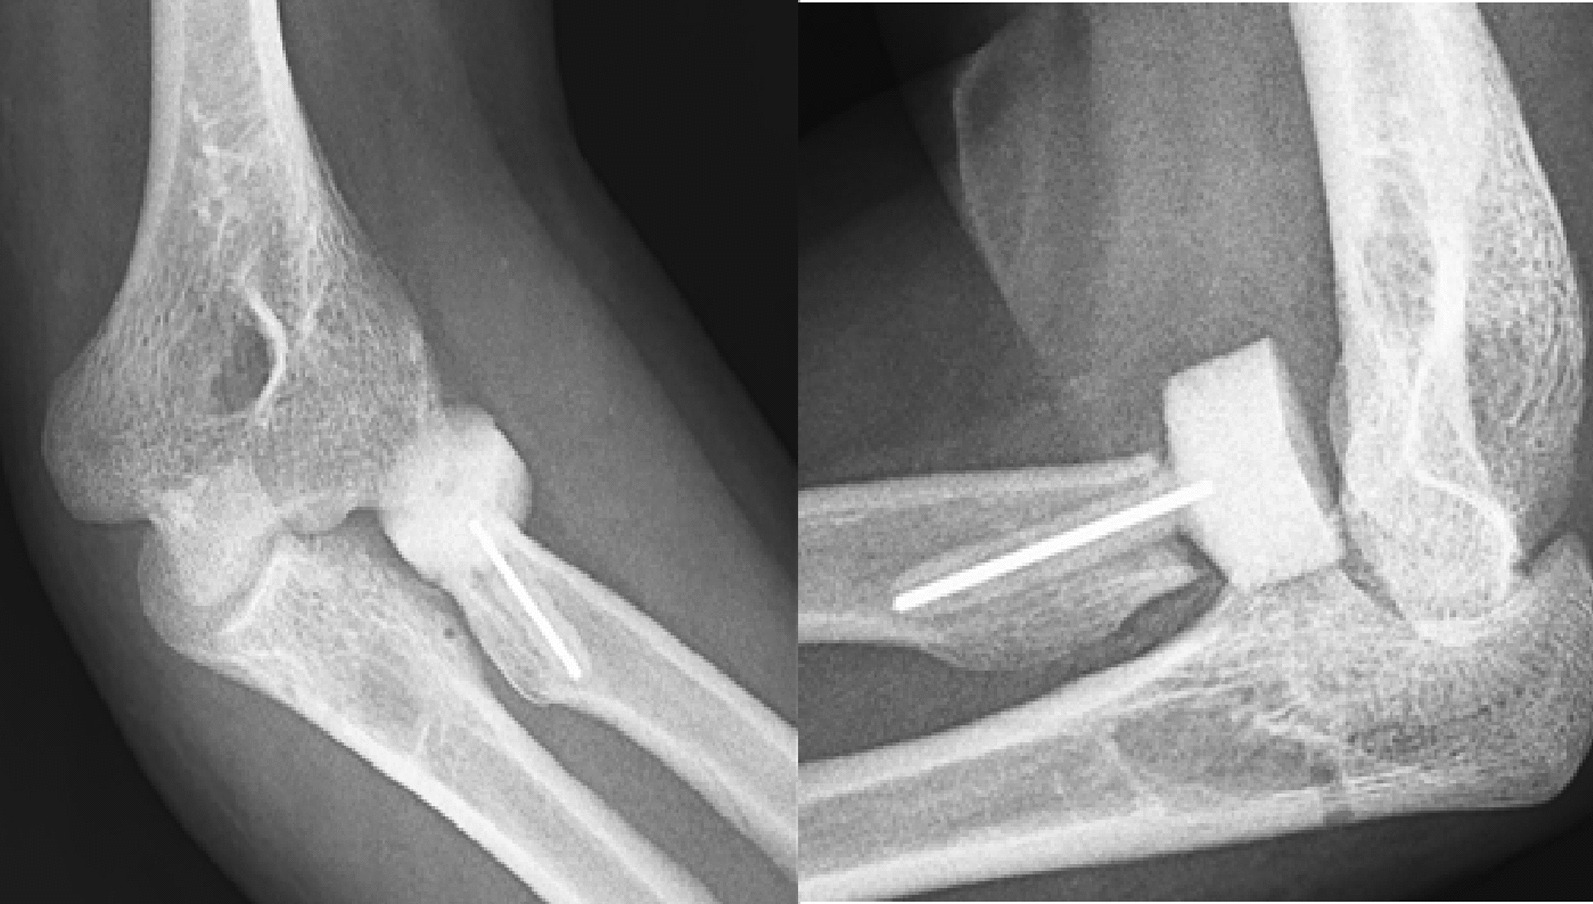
Radiographic images of left elbow in follow-up month 6; the joint is reduced and congruent, and no indications of spacer impairment or osteolysis in the proximal radius have been observed
